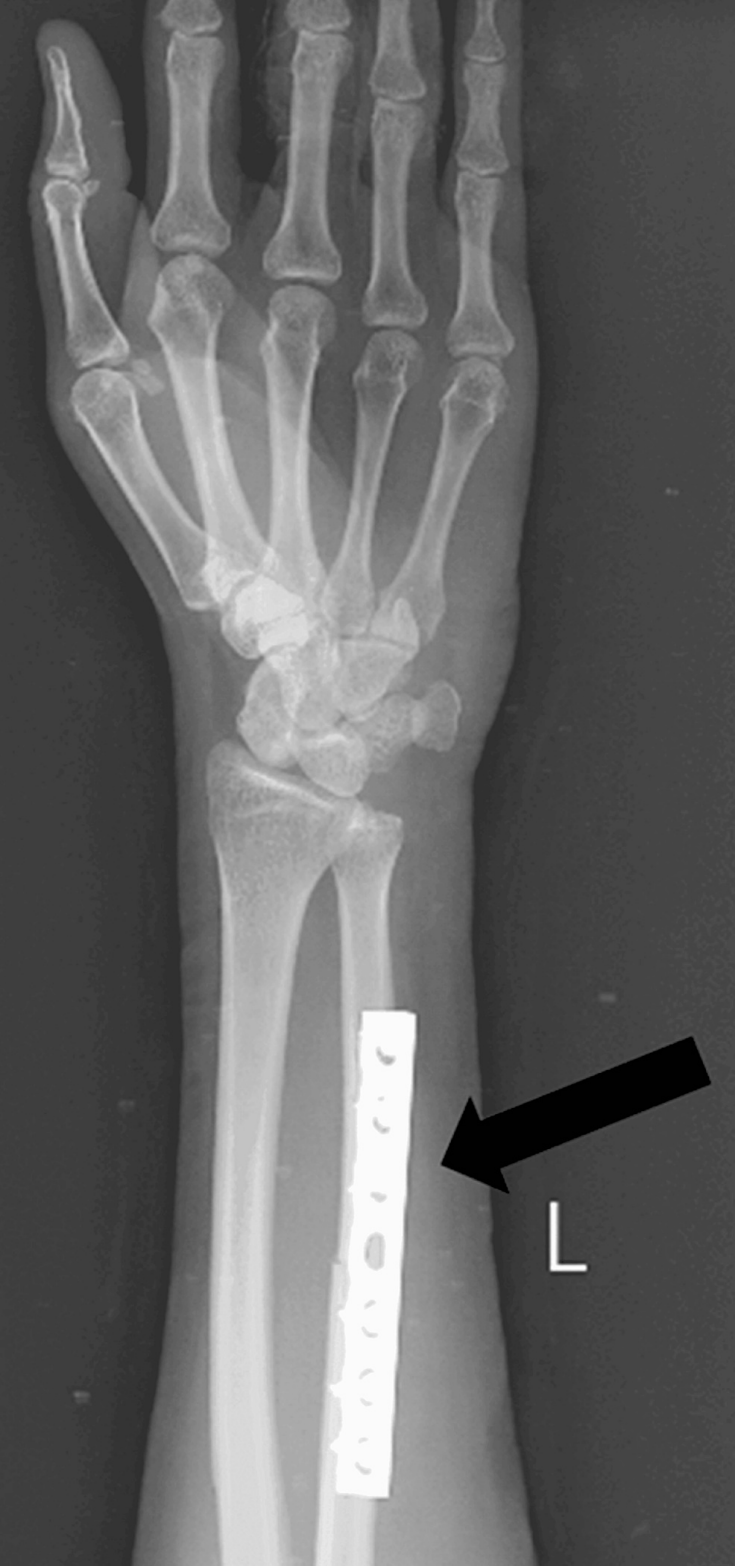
Post-operative X-ray of the left forearm The arrow represents an isolated ulnar shaft fracture managed by open reduction and internal fixation with plate osteosynthesis.
